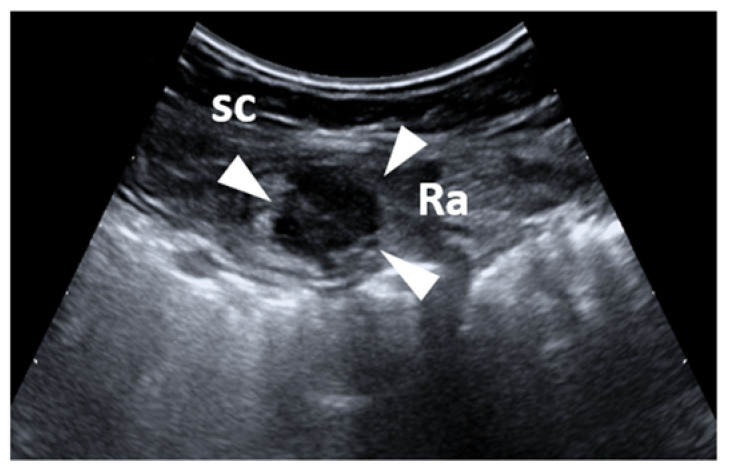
Short-axis sonogram with a convex transducer shows a hypoechoic, nodular mass (white arrow), located on the peritoneal superface, and inside the rectus abdominis (Ra) muscle. sc: subcutaneous tissue.
